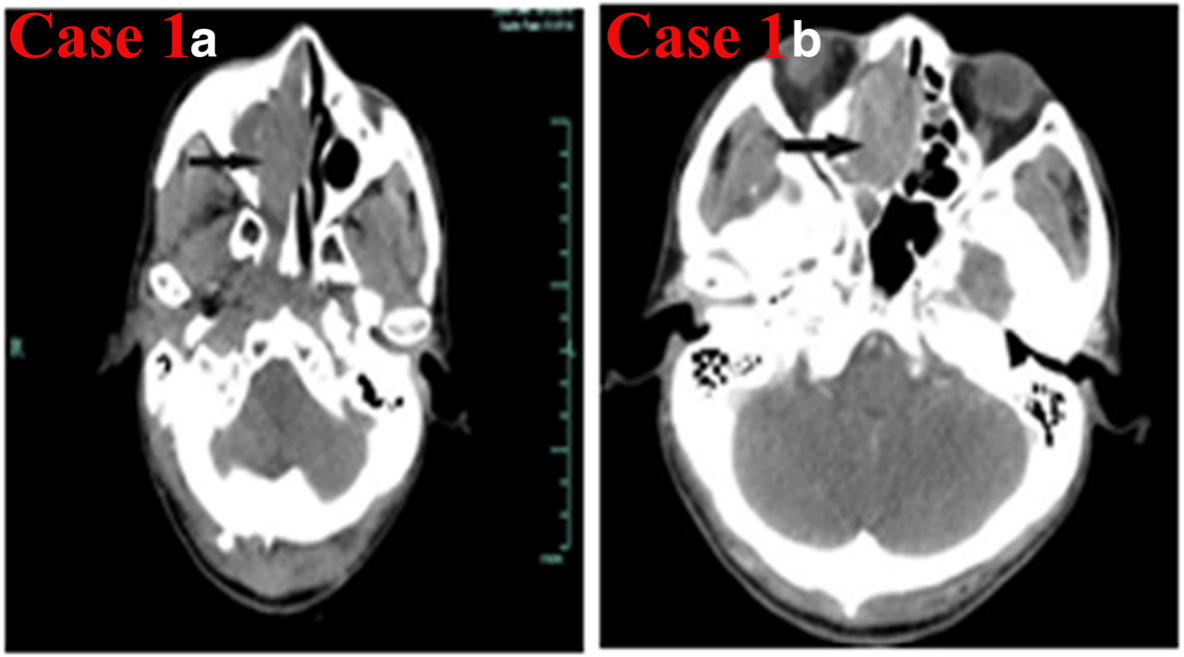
CT of the sinonasal small-cell neuroendocrine carcinoma of Case 1. a) Noncontrast axial CT showing a soft-tissue mass in the right maxillary sinus involving the right nasal cavity, right ethmoid sinus, and sphenoid sinus. b) Contrast-enhanced CT showing that the lesion was strongly enhanced.
